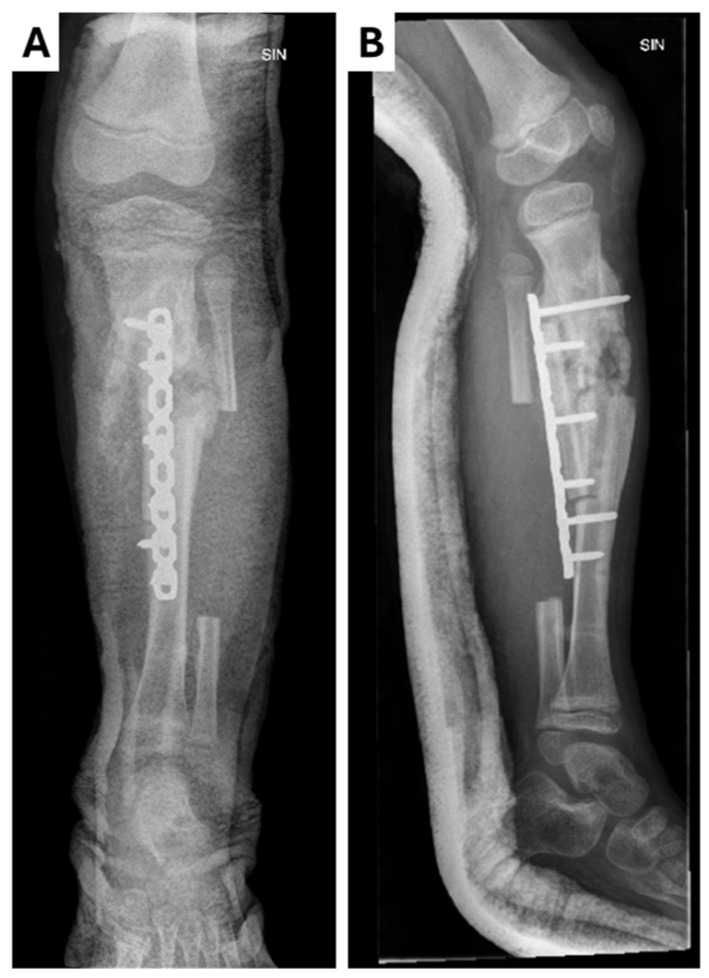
Radiological examinations of the left leg showing chronic osteomyletic changes in the tibial diaphysis in the (A) X-ray anterior–posterior projection; (B) X-ray lateral projection. A proximal–distal osteotomy of the tibia was performed, resecting the pseudoarthrosis and exposing the bone surfaces. A proximal–distal osteotomy of the fibula was also performed, along with the rotation of the bone fragments to place them in the defect around the pseudarthrosis. Osteosynthesis was performed with a locked plate and five screws while maintaining the axis of the bone. A sterile aseptic dressing and plaster cast were applied.
